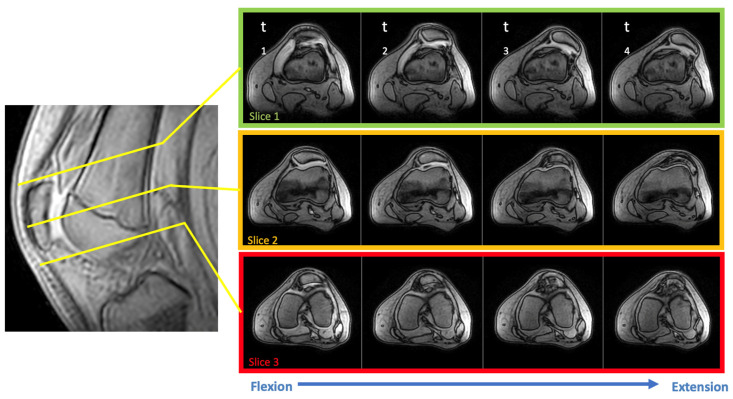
Real-time dynamic MRI measurement of patellar maltracking in the left knee of a symptomatic patient. In accordance with the technique described by Frings et al. [5], three slices in transverse orientation were followed by one sequence in sagittal plane orientation. Four measurement time points (t_1_–t_4_) are shown. During active repetition of knee flexion and extension, patellar tracking could be measured in real time.
